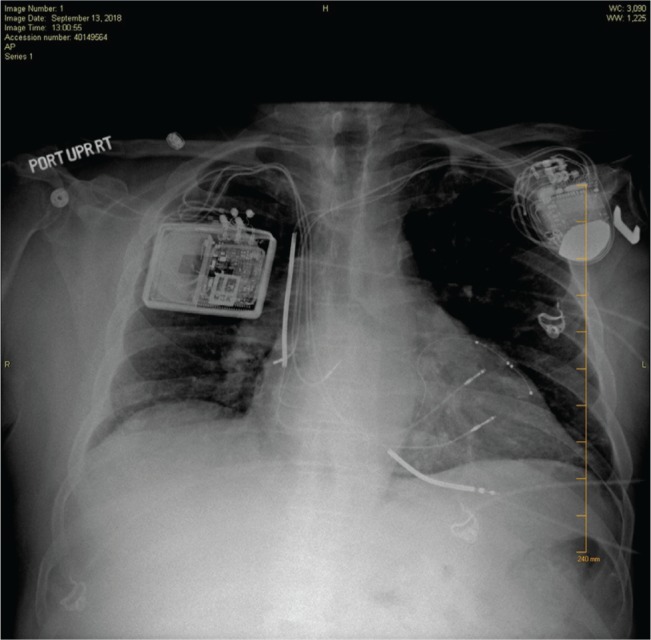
Preprocedure chest radiograph revealing CRT-D and the Optimizer™ III device (Impulse Dynamics, Orangeburg, NY, USA).
